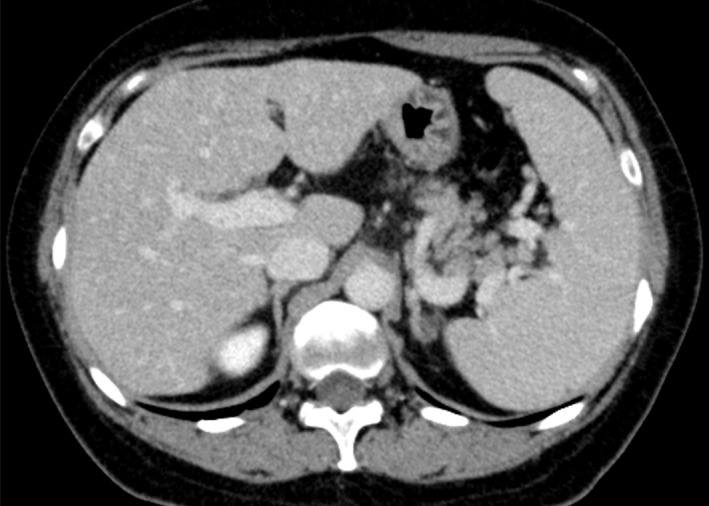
Abdominal computed tomography scan showed splenomegaly without any other abnormal finding
